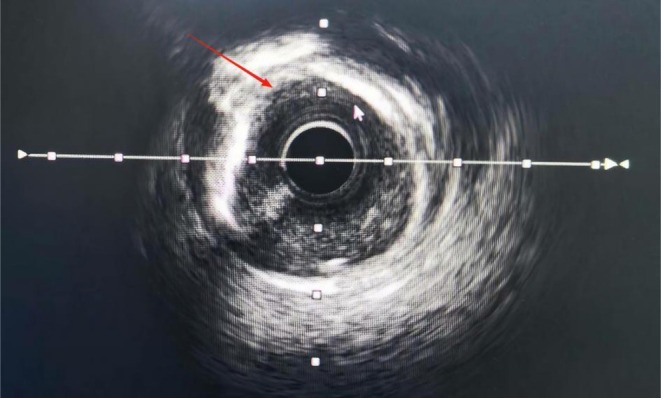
Intravascular ultrasound image showing fragmentation of calcified plaque following intravascular lithotripsy.
